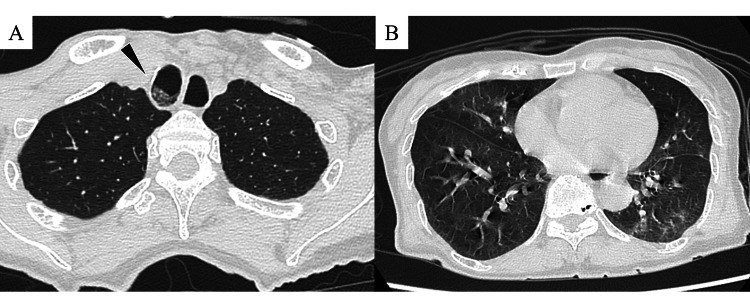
Chest computed tomography without contrast enhancement. Chest computed tomography without contrast enhancement showed intrabronchial fluid retention (arrowheads, A) and ground-glass opacities in the right middle lobe, left upper lobe, and lower lobe (B).
